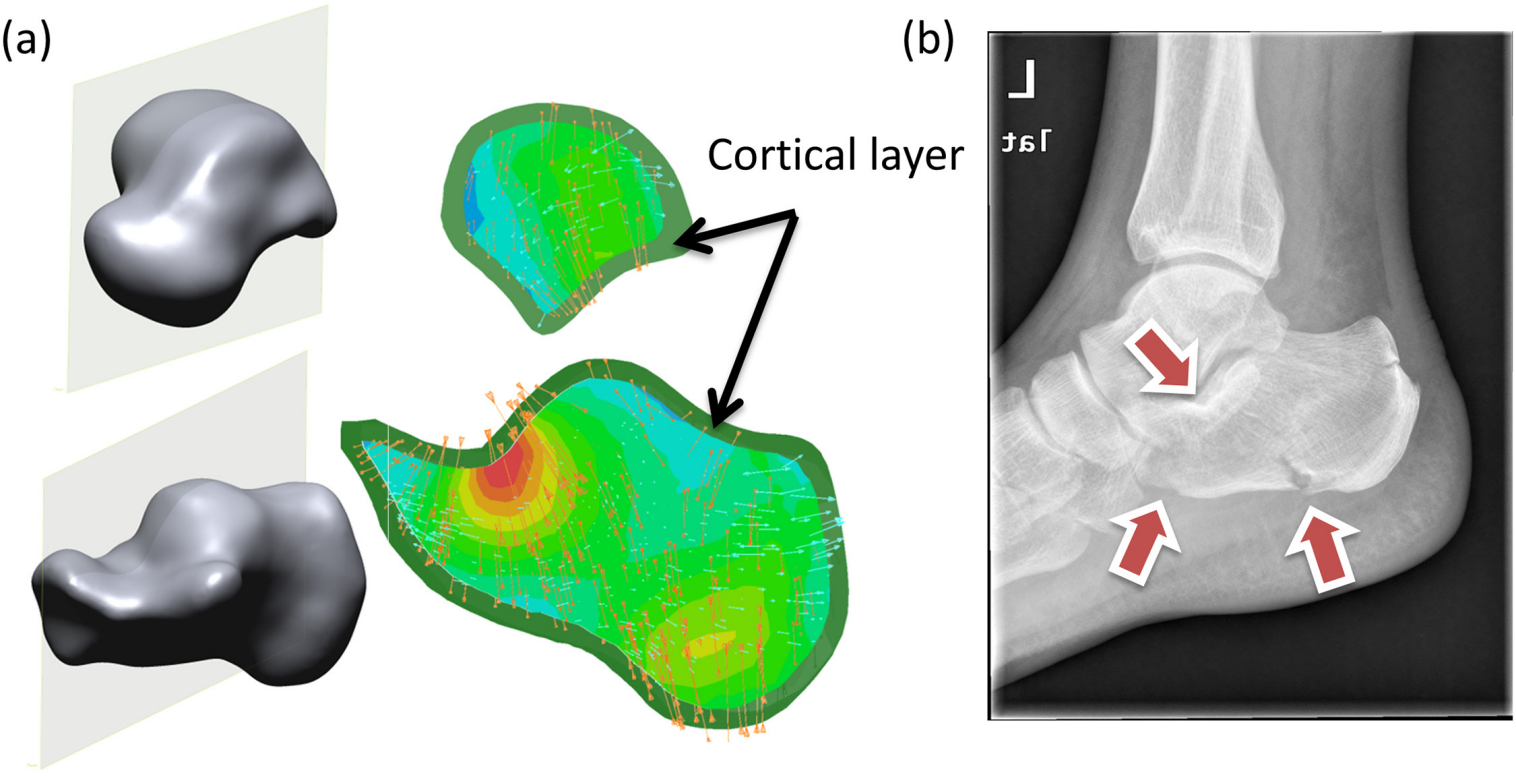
Von Mises stress of calcaneus and talus. (a) Cross section view of von Mises stress of the calcaneus and talus at 5.0 m/s impact velocity. Orange arrows indicate compressive stress. Cyan arrows indicate tensile stress. (c) X-ray of a typical patient with calcaneus fracture due to high compressive load. Arrows indicate regions of fractures.
